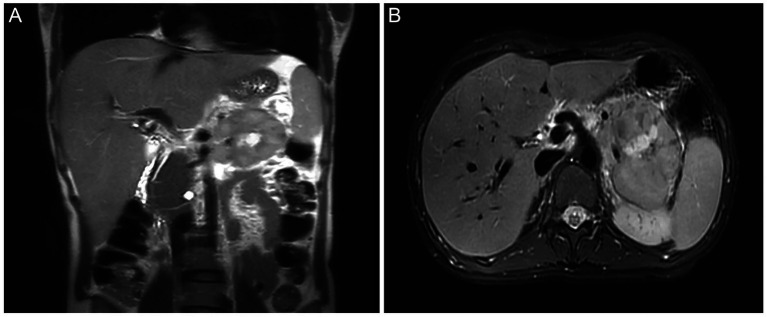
Radiological images. MRI scans showed an occupying lesion involving the tail of the pancreas (A: coronal view; B: axial view).
